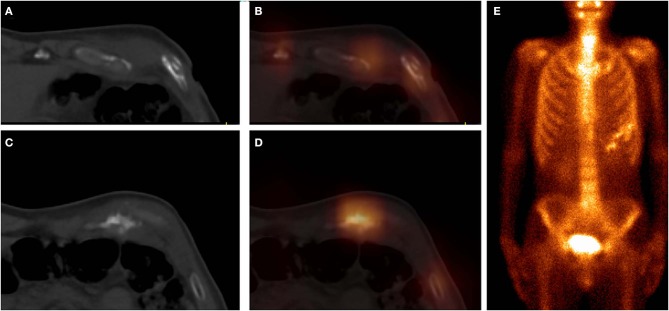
These images were obtained from a 59-year-old patient of CG, who underwent chemotherapy and surgery for esophageal cancer 12 months ago. ^99m^Tc-MDP SPECT/CT axial images (A, CT; B, fusion; C, CT; D, fusion) and WBS image (E, anterior) demonstrated high-uptake tracer activity in the seventh costal cartilage, with irregular calcification and adjacent soft tissue nodules. The pathological findings confirmed the diagnosis of costal cartilage invasion by chest wall metastasis.
